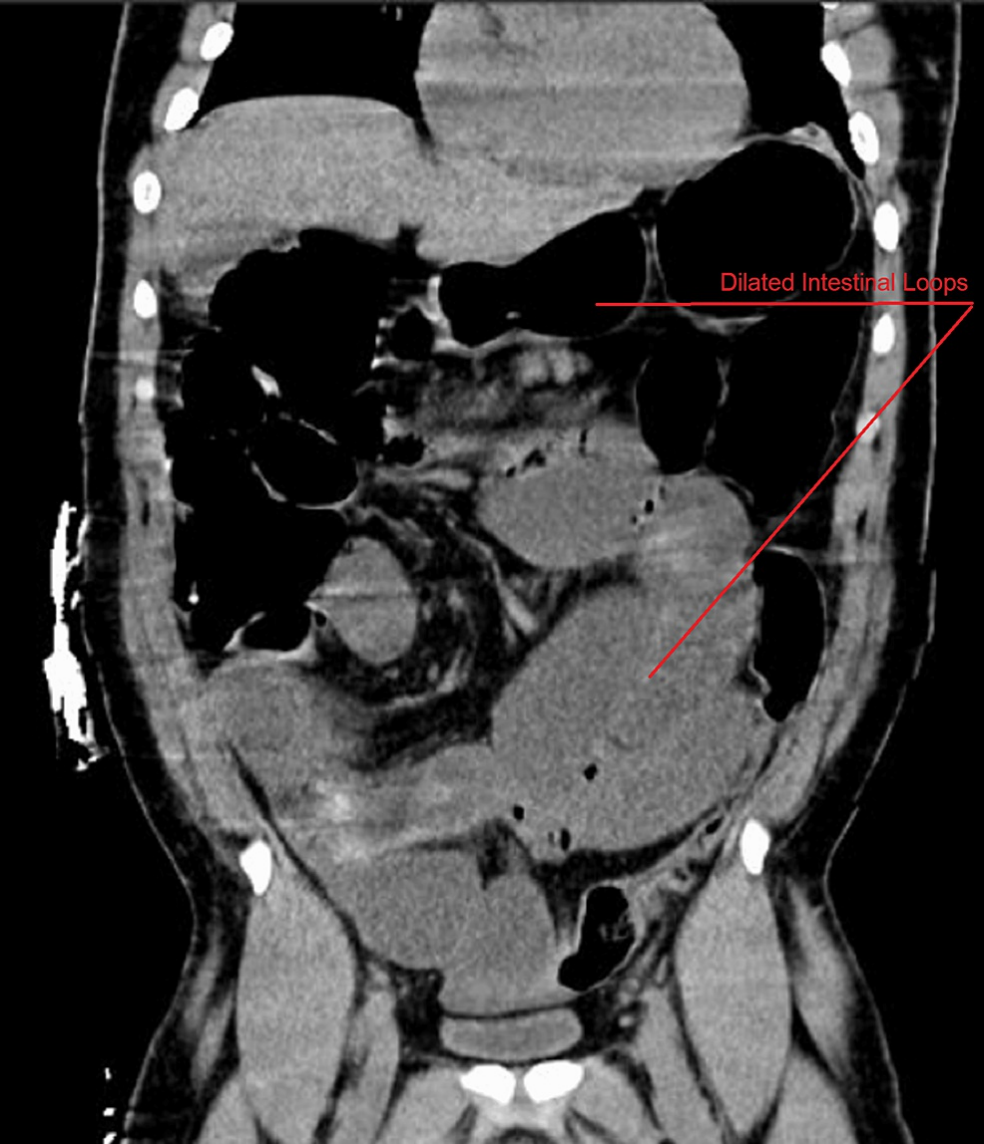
Abdominal CT scan with contrast showing distal small intestine obstruction with the swirling of mesenteric vessels.
